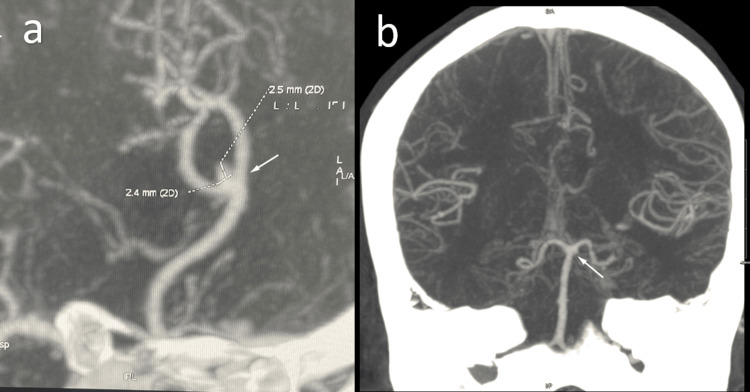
Brain computed tomography angiography (CTA) Small aneurysm at the bifurcation of the azygos anterior cerebral artery (ACA) measuring 2.5 mm in diameter (a,b)
